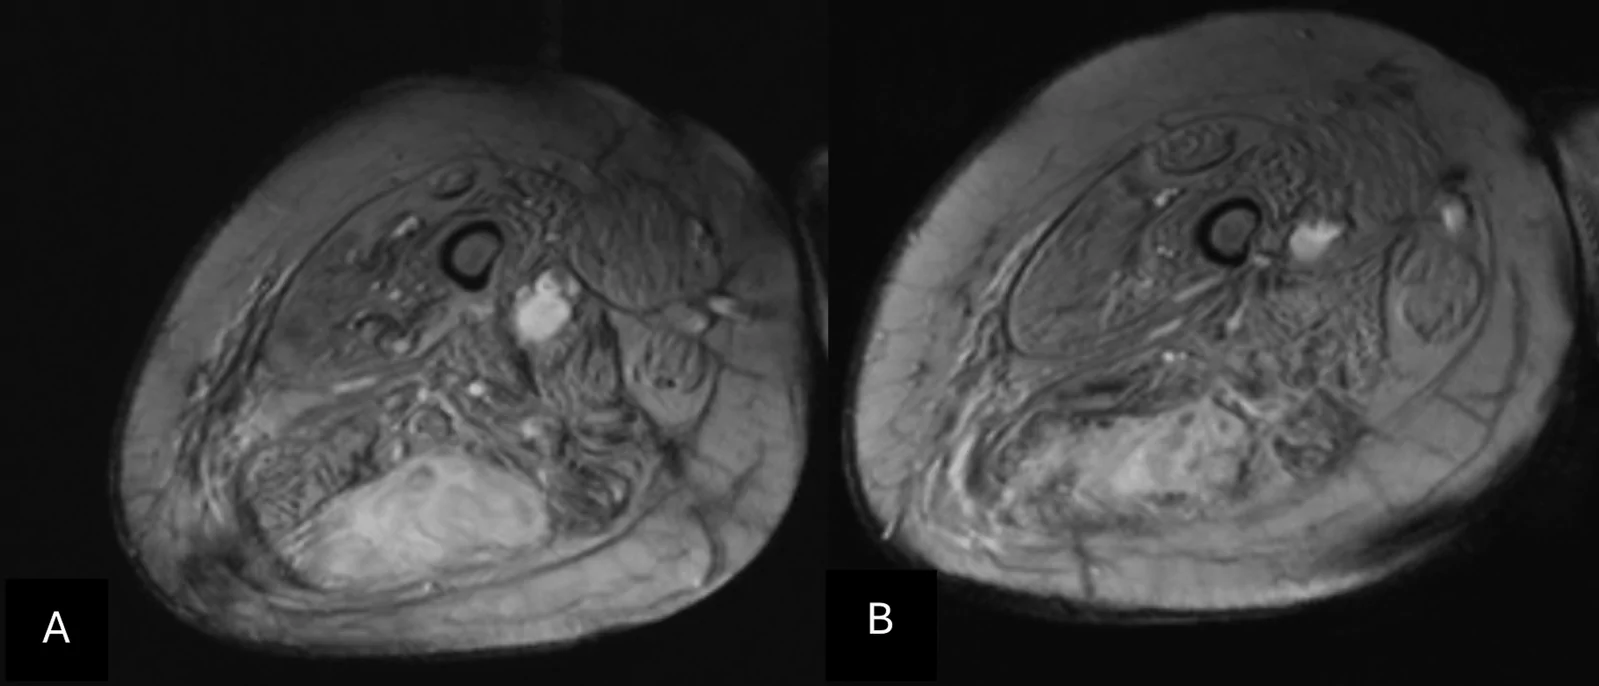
Preoperative MRI of the thigh. A and B show a large plexiform over the posterior thigh compartment, measuring 6.5 x 3.3, and a heterogeneous mass arising from the distal sciatic nerve, measuring approximately 3.5 x 3 cm, in the axial plane, starting from the mid-thigh down to below the knee.
